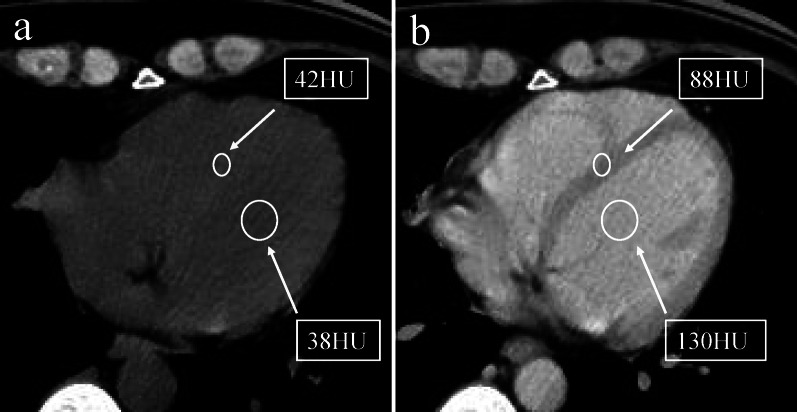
Region of interest for extracellular volume calculations on computed tomography in a 55-year-old woman with breast cancer. a Contrast-enhanced scan, the ROIs were manually delineated in the middle of the interventricular septum and blood pool avoiding the papillary muscle. b Unenhanced scan, copy the two ROIs in the contrast-enhanced image and place them at similar locations in the unenhanced ventricular septum and blood pool. *ROI*, region of interest
